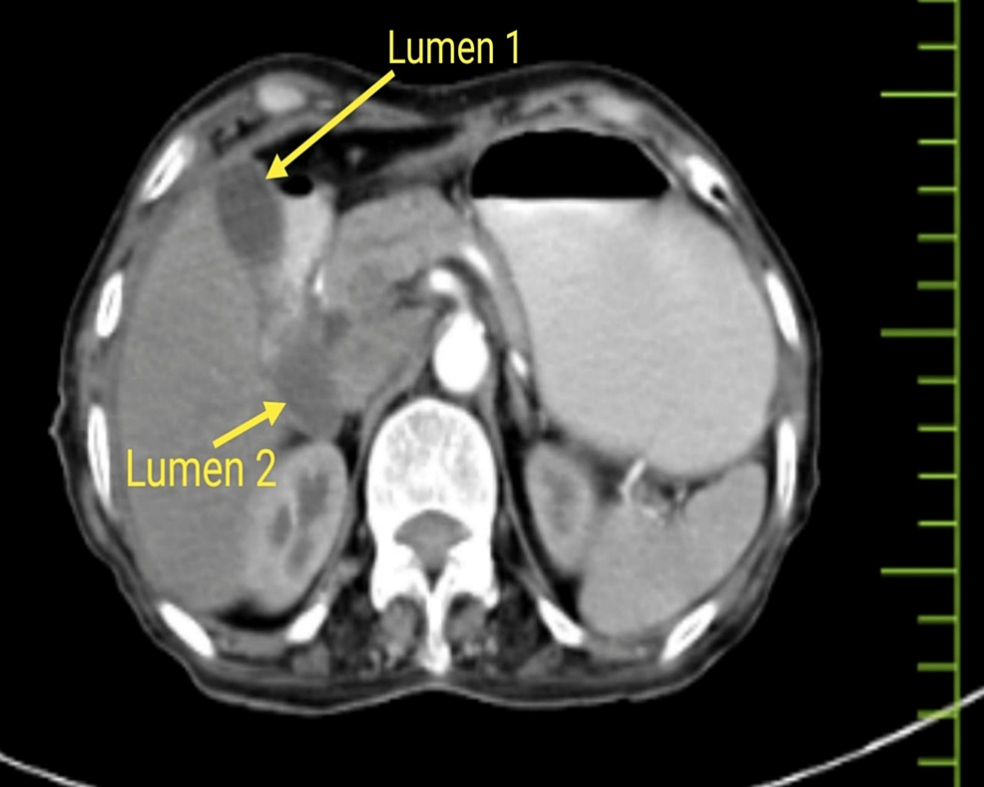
Computed tomography showing anomalous gallbladder
